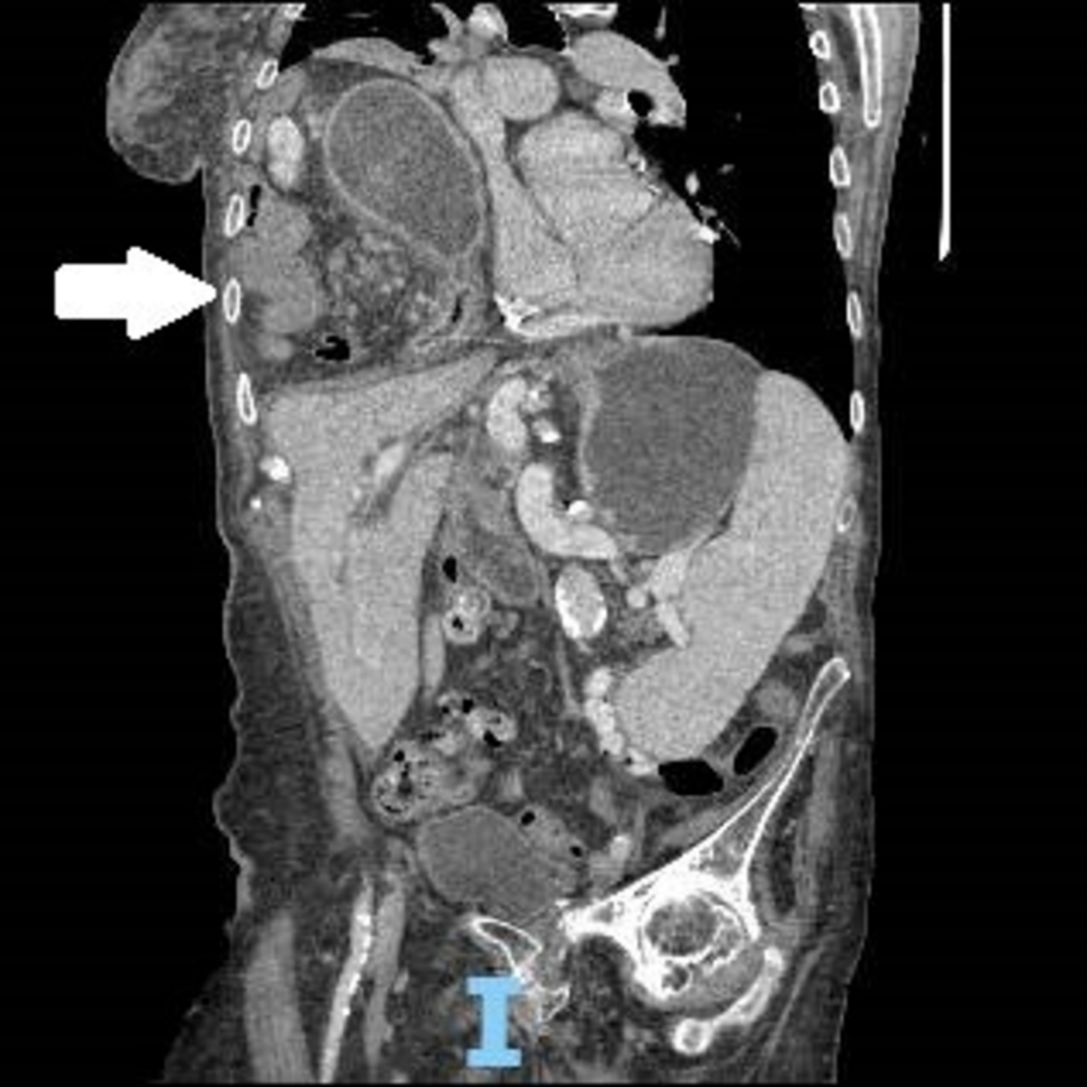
Coronal view computed tomography on admission; arrow indicating stomach and intestinal contents in the thoracic cavity
